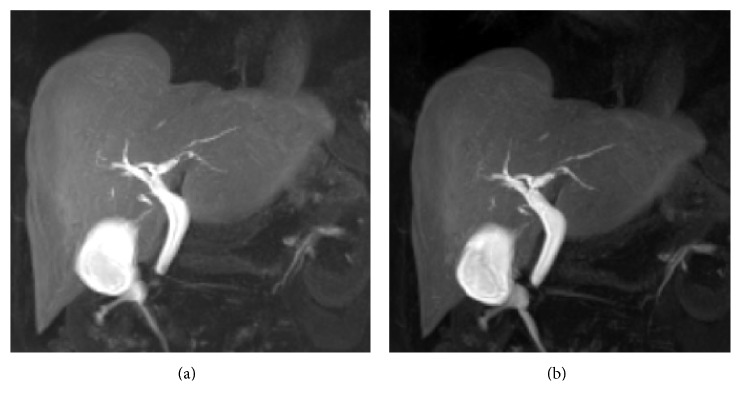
Influence of flip angles. 3D T1-weighted spoiled gradient echo sequences, with different flip angles (10° in (a), 30° in (b)). Delineation of the biliary ducts is better increasing the flip angle, depicted in (b) as well. The increase of flip angle improves the SNR and the CNR; a flip angle >20° is generally recommended.
